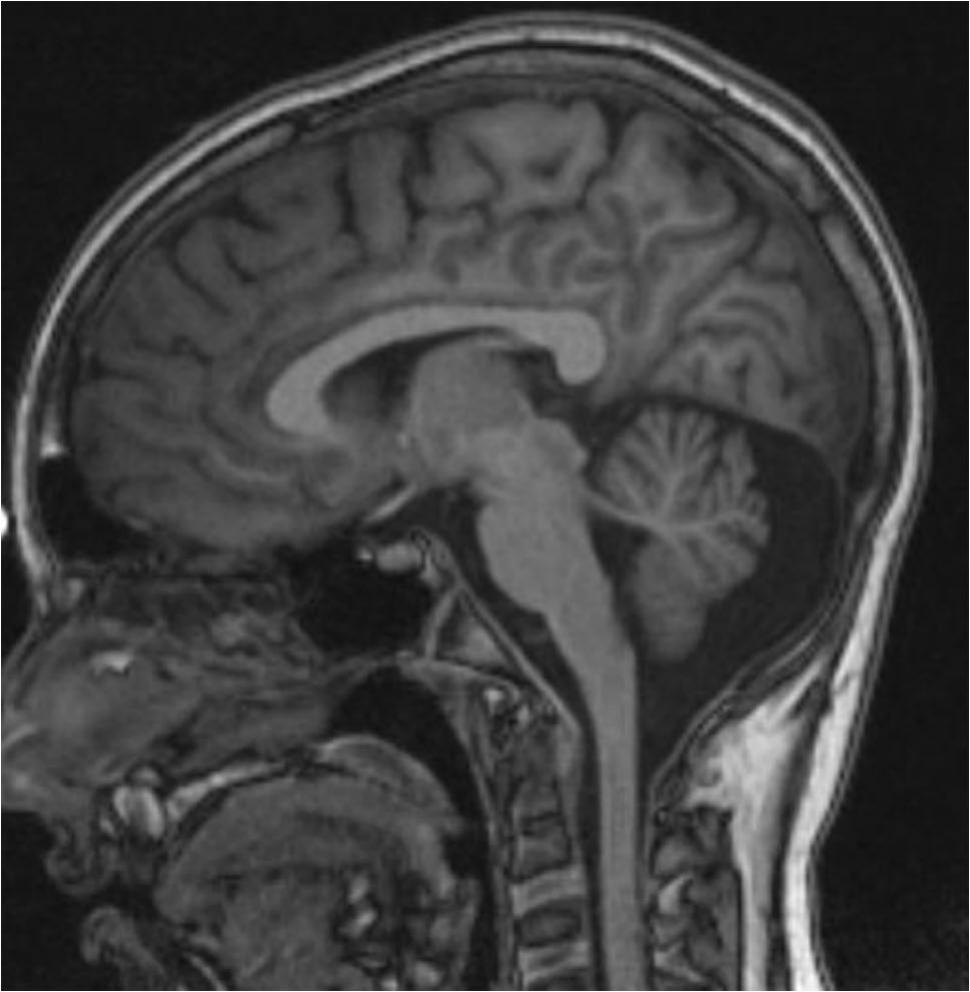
Sagittal T1-weighted cerebral MRI scan showing vermian hypoplasia
